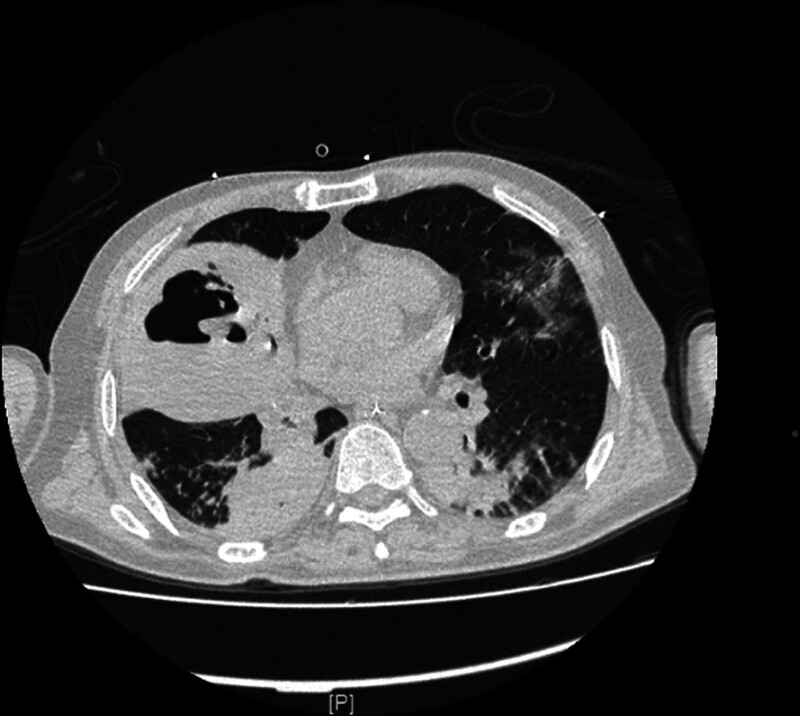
Chest CT revealed consolidation of the right middle and lower lobes (cavities with a gas–liquid interface inside were found within the lesion in the middle lobe).
